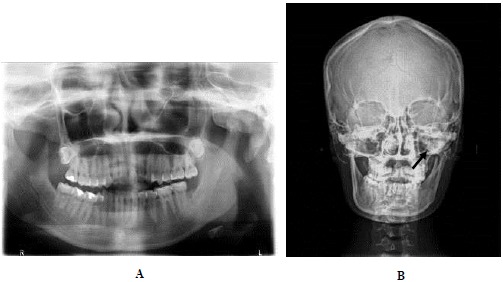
A. Panoramic radiograph showed a radiopaque area on the left condylar region with similar density to the adjacent bone. B. PA skull showed a well defined bony overgrowth extending medially from the left condyle, facial asymmetry and deviation of mandible to the right side.
